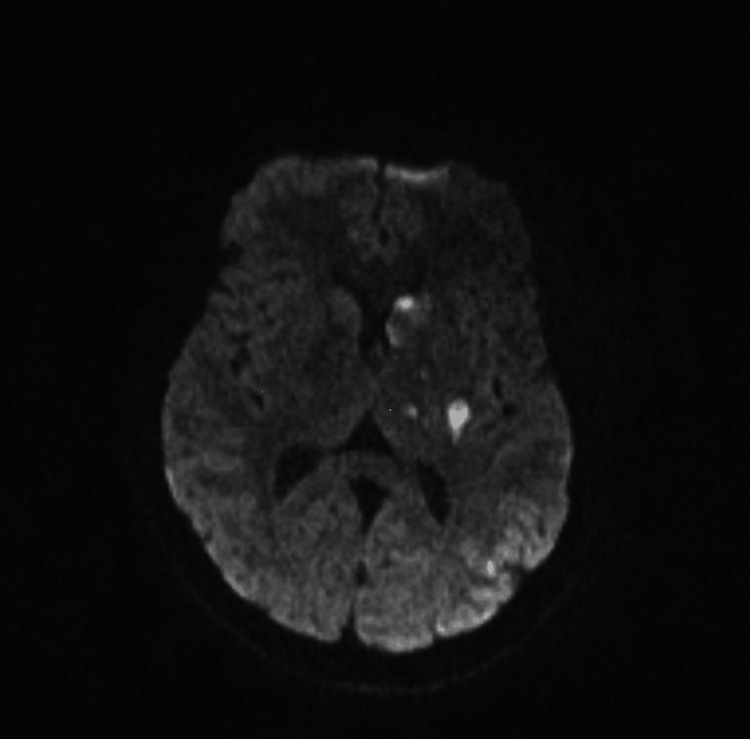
MRI brain showing multiple acute non-hemorrhagic infarcts involving the left cerebral hemisphere with features suggestive of raised intracranial tension in the left frontal region
